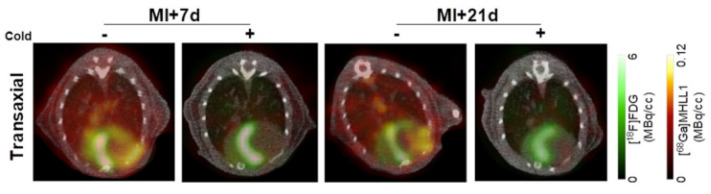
Fibrosis imaging: PET/CT fusion transaxial images at 7 d and 21 d after myocardial infarction with and without co-administration of unlabeled ligand. Accumulation of fibrosis tracer [^68^Ga]MHLL1 (orange) in the non-viable infarct territory defined by [^18^F]FDG (green) with additional distribution at the site of the surgical wound [52].
